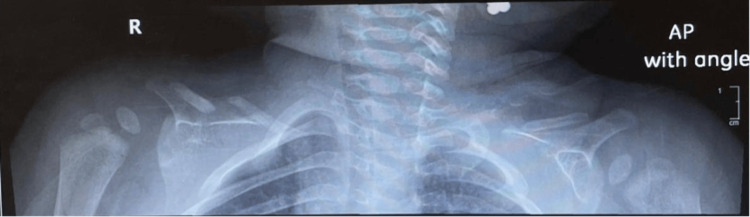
Bilateral Clavicle X-ray During Out Patient Department Follow-Up.
